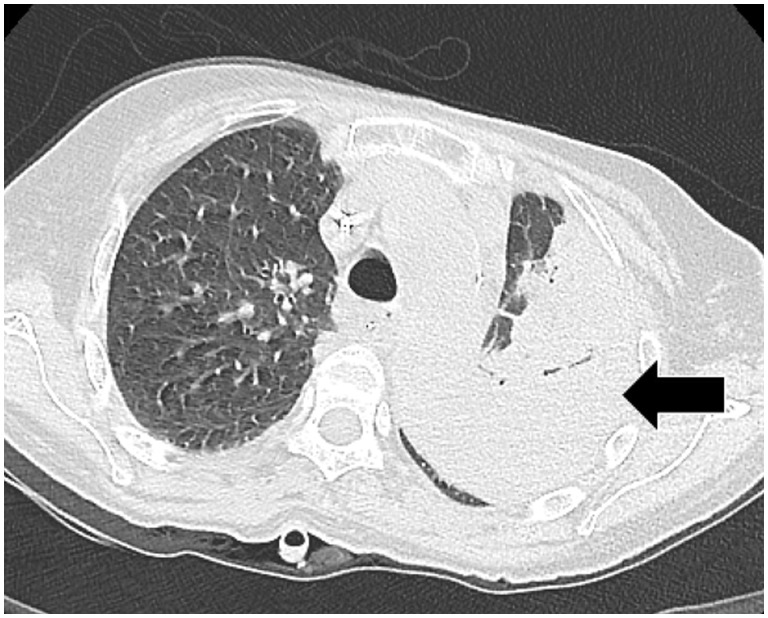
Pneumonia. Axial CT scan lung window section showing left lung consolidation (arrow). Sputum culture demonstrated S*taphylococcus aureus.*
